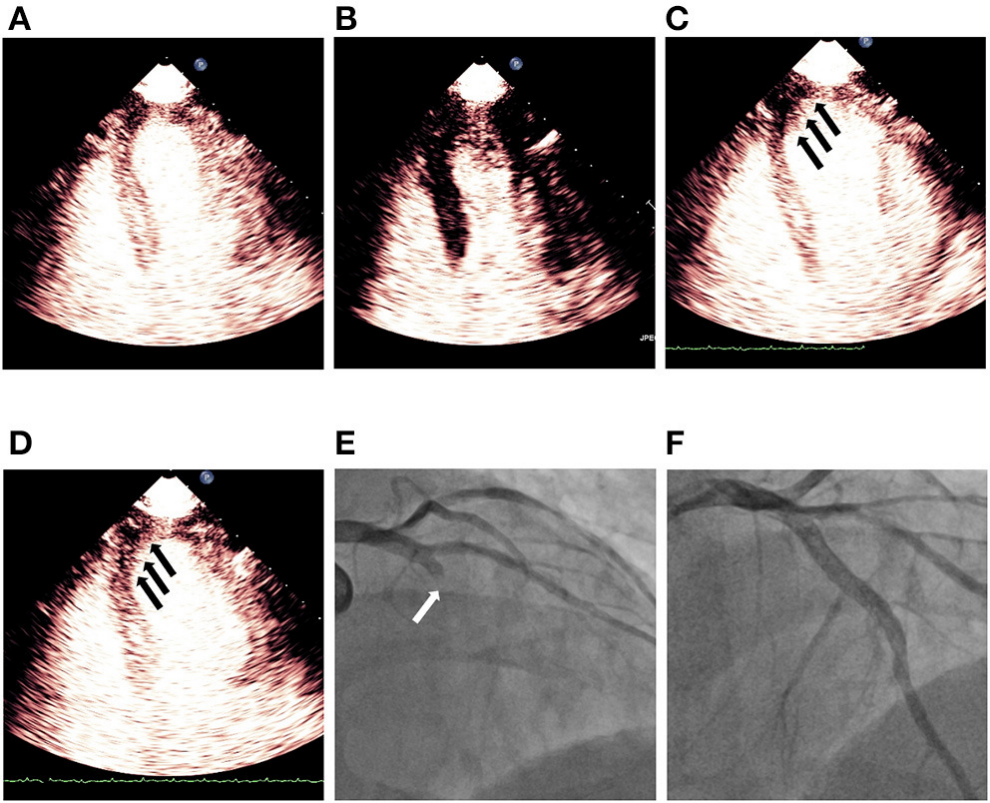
Demonstration of persistent no reflow phenomenon noticed on MCE after successful revascularization of LAD STEMI. (A) Shows myocardial contrast replenishment before the high MI impulse, while (B) indicates complete microbubble destruction immediately after the high MI impulse. (C) Shows a defect (*black arrows*) in the segments of the infarct zone during replenishment (~4 beats post-high MI impulse), while (D) indicates a persistent defect (*black arrows*) in LAD territory although a plateau intensity (~10 beats after the high MI impulse) had been reached. (E) Shows blocked LAD (*white arrow*) before PCI and (F) indicates revascularization of LAD after successful PCI. LAD, left anterior descending; MCE, myocardial contrast echocardiography; PCI, percutaneous coronary intervention; STEMI, ST segment elevated myocardial infarction.
